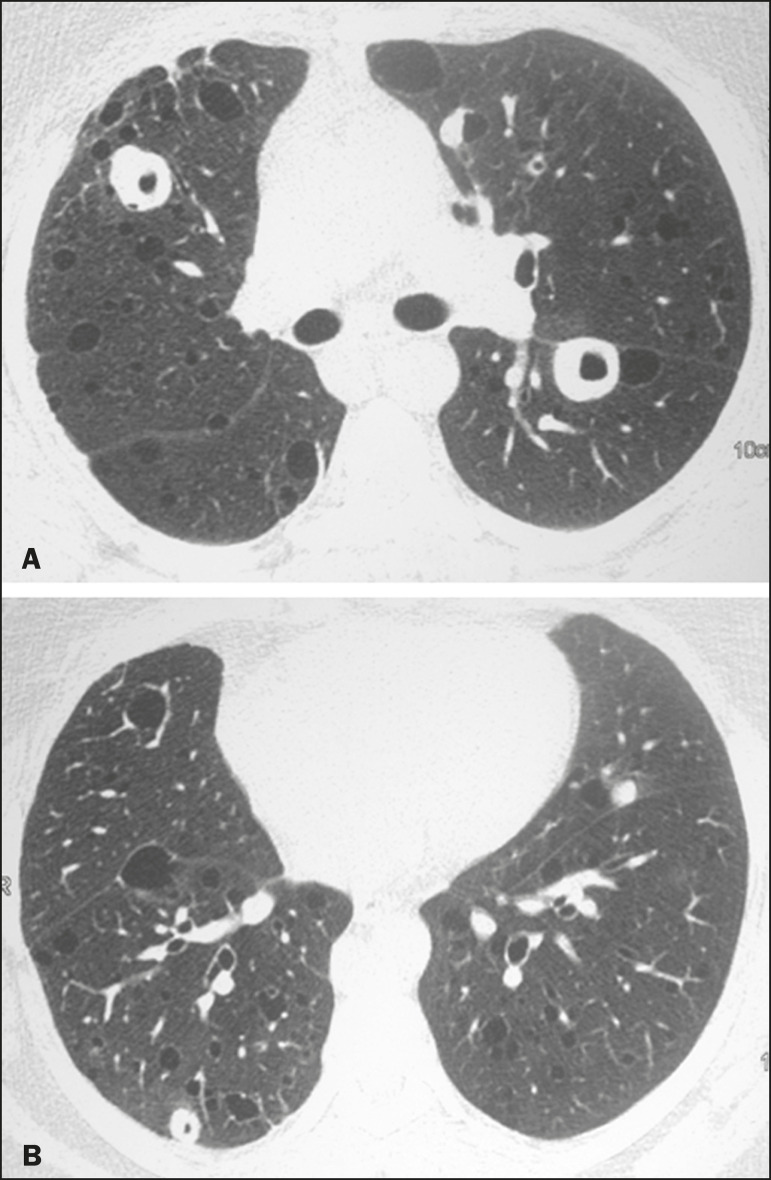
A 50-year-old woman with LIP related to amyloidosis. Chest CT scans show multiple cavitated nodules associated with pulmonary cysts.
